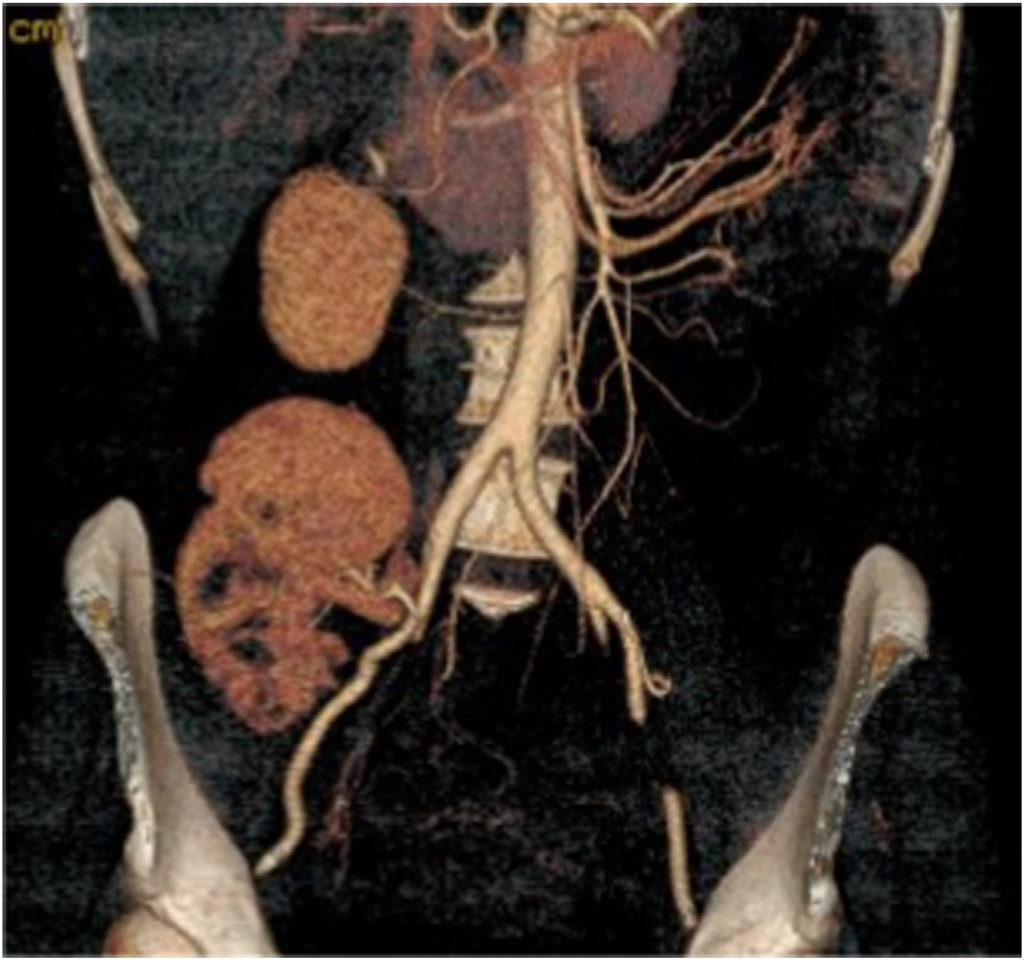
Postoperative computed tomography angiogram demonstrating autotransplanted kidney with patent renal artery and stable mid- and inferior pole infarct.
